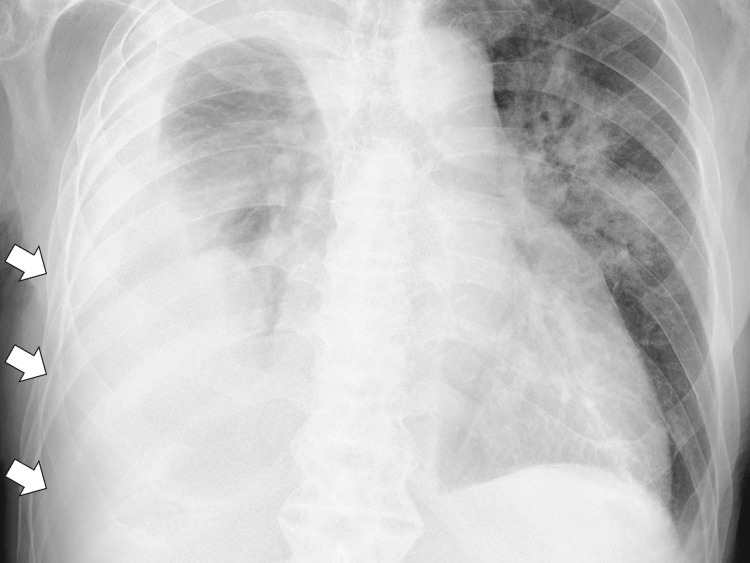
Chest X-ray showing bilateral infiltrating shadows and right pleural effusion (white arrows)
